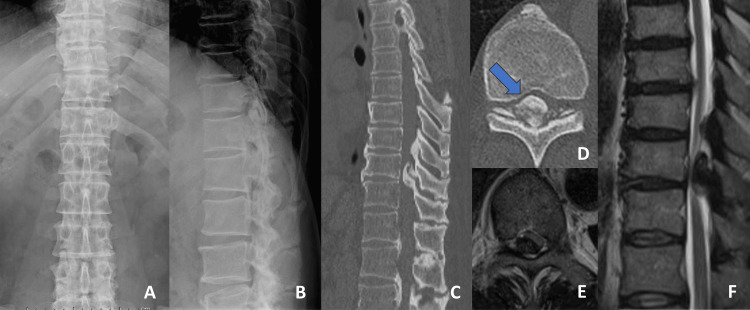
Preoperative X-ray, computed tomography (CT), and magnetic resonance imaging (MRI). (A) X-ray: anteroposterior view. (B) X-ray: lateral view. (C) Sagittal CT. (D) Axial CT: severe OLF with an occupation rate of 81%, classified as the tuberous type (arrow). (E) T2-weighted axial MRI. (F) T2-weighted sagittal MRI.
